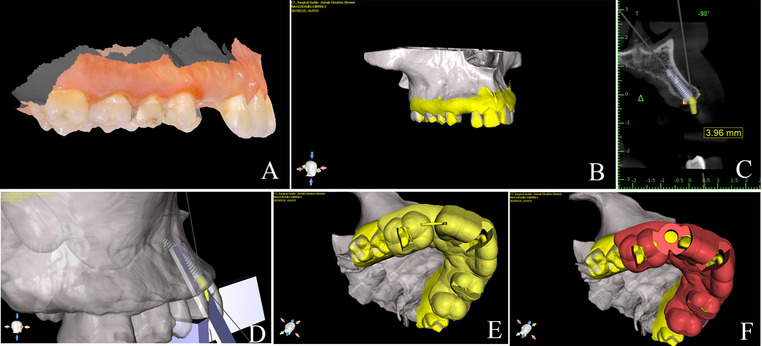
Preoperative digital treatment planning for an osteoperiosteal flap case. A STL file of intraoral scan. B CBCT segmentation and superimposition with intraoral scan. C Bone width measurement and implant size selection. D Osteotomy vector determination. E Osteoperiosteal flap surgical guide design. F Implant placement surgical guide design. CBCT = Cone Beam Computed Tomography; STL = Standard Tessellation Language
